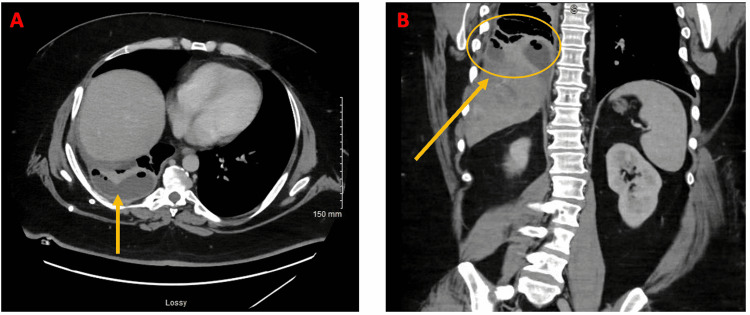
CT of the abdomen/pelvis with contrast on hospital day eight (second admission) (A) Axial view of loculations in empyema. (B) Coronal view of loculations in empyema.
